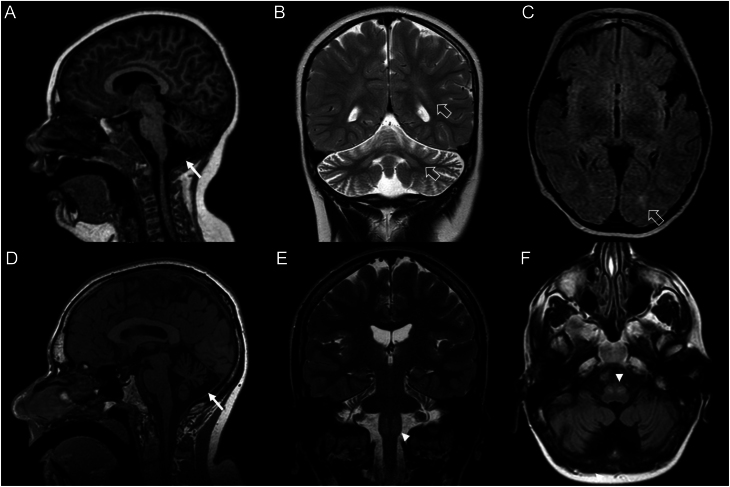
Brain MRI images: A, B, C patient n1, D, E, F patient n2. (A, D) sagittal T1-weighted image; (B, E) coronal T2-weighted image; (C, F) axial FLAIR T2-weighted image. The study shows cerebellar atrophy (white arrow), cerebral atrophy with widening of the Sylvian fissures, bilateral superior olivary nuclei increased signal on FLAIR and T2-weighted images (arrowhead), and posterior parietal and cerebellar white matter hyperintensity on FLAIR and T2-weighted images (empty arrow).
